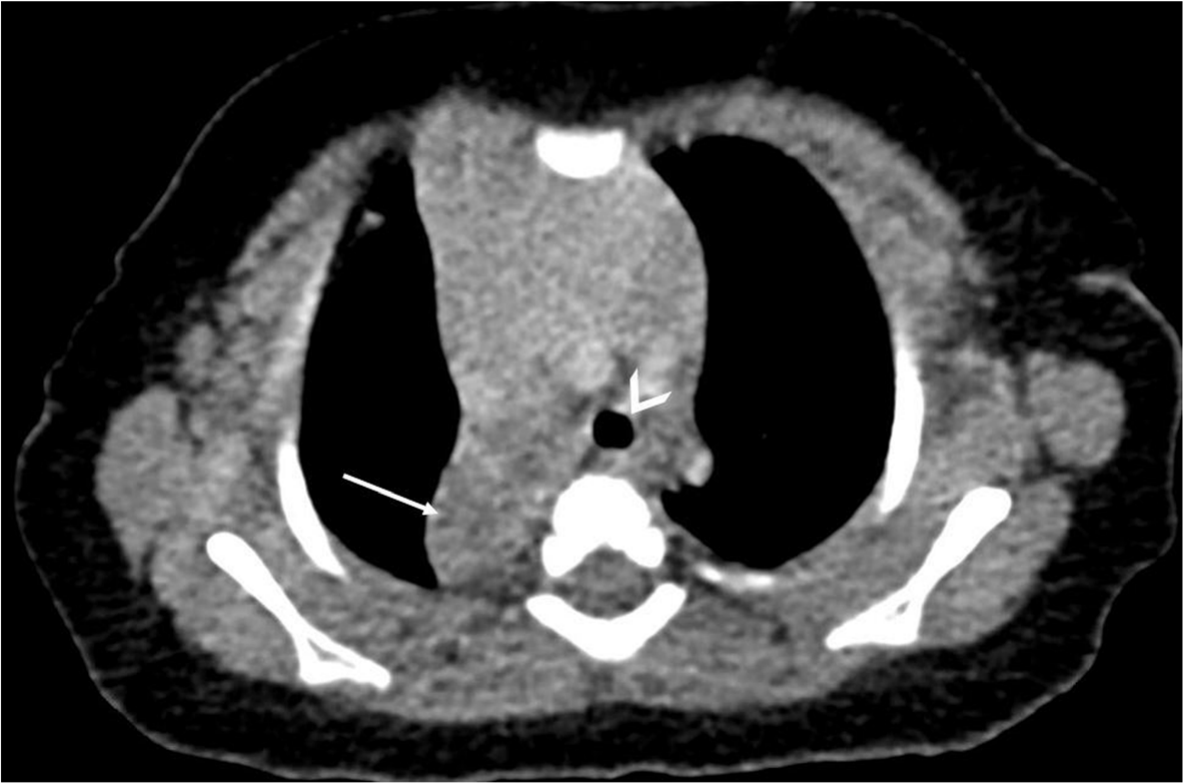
Non-contrast-enhanced axial CT post-aspiration of the mediastinal mass shows significant improvement in the mass effect on the trachea (arrowhead) and thymus. The right upper posterior consolidation concerning pulmonary sequestration (arrow) remains unchanged
